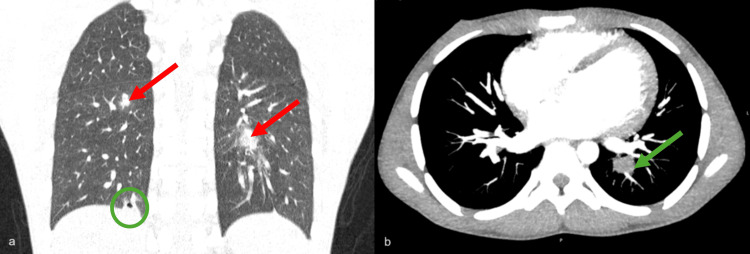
Thoracic CT scan (a) Coronal axis - nodular images consistent with septic emboli in the left mediobasal segment and in the upper segment of the right lower lobe (red arrows). Also visible is a cavitated nodule in the right posterobasal segment (green circle); (b) Axial axis - a septic embolus, measuring 15 mm, is seen in the left mediobasal segment, accompanied by endoluminal filling of two segmental arterial branches (green arrow).
